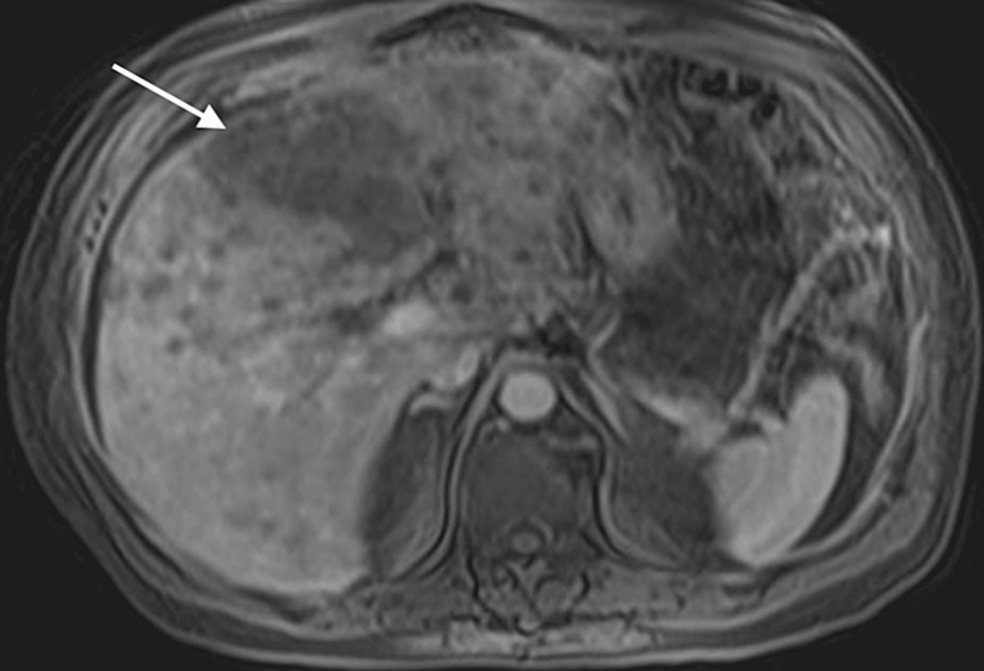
CT abdomen (Case 3) showing large central hepatic mass 8.4x8.4x3.2 cm with numerous hypoenhancing masses throughout liver.
